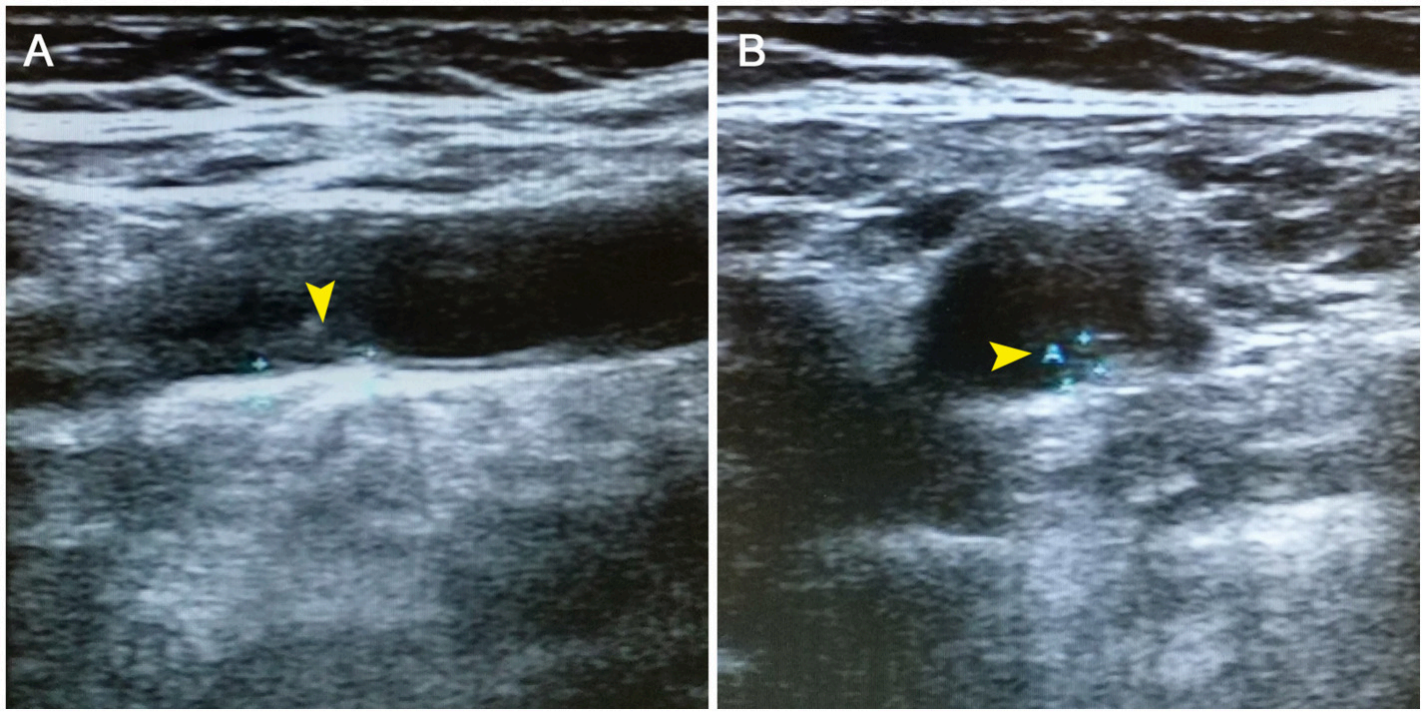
Cervical color doppler ultrasonography revealing atherosclerotic plaques (A,B, arrowheads) of the left internal carotid artery.
